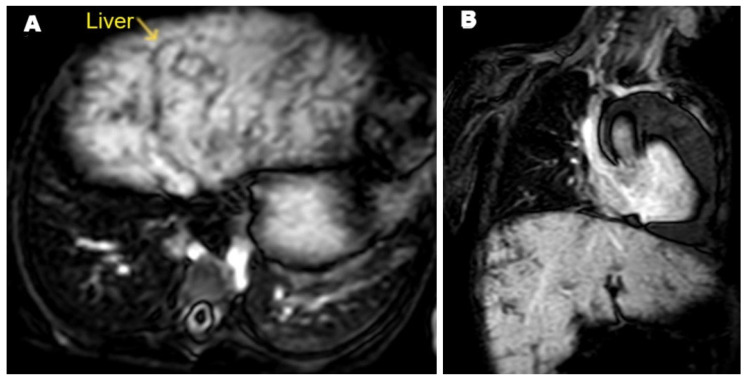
Selected late gadolinium enhancement (LGE) MRI images. (A) Axial and (B) coronal sections showing contrast reflux into the hepatic veins, causing nutmeg appearance of the liver and confirming the diagnosis of Budd–Chiari syndrome.
